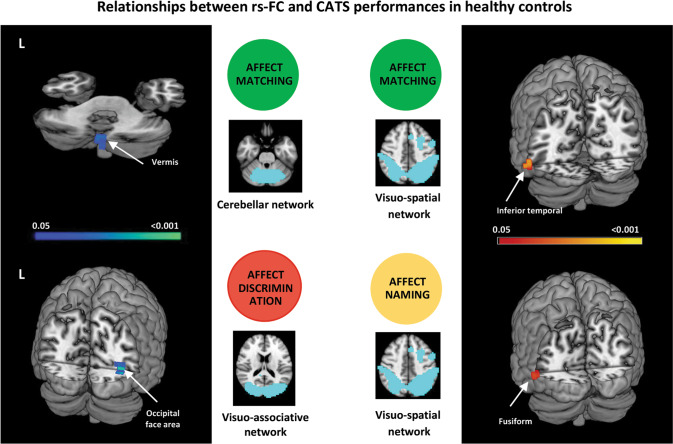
Independent component analysis. Relationship between resting state functional connectivity and CATS scores in healthy controls. Positive and negative relationships are shown in cold and warm colors, respectively. Results are overlaid on the Montreal Neurological Institute (MNI) standard brain and displayed at *p* < 0.05 Family-wise error corrected for multiple comparisons. Age at MRI, sex, education and gray matter density were included in the model as nuisance variables. rs-FC resting state functional connectivity, L Left. Color bar represents *p* values.
